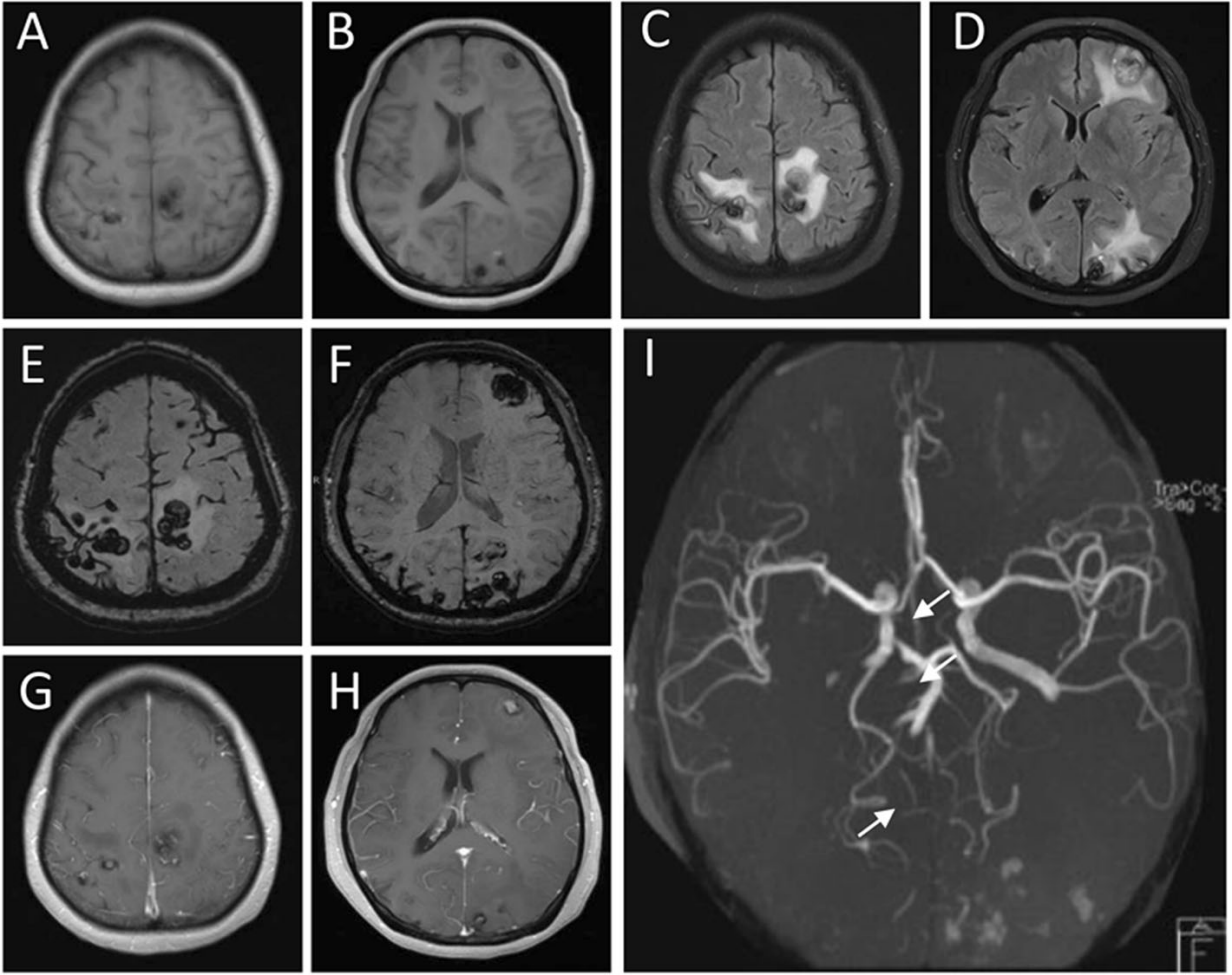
MRI brain images show multiple brain metastases of myxoma, hemorrhage and cerebral aneurysm formation from myxoma. a-b The axial T1-weighted images. c-d The axial T2-weighted flair images show multiple nodules or mass shadow. e-f The axial SWI images shows multiple cerebral hemorrhage. g-h Magnetic resonance enhanced scan showed heterogeneous reinforcement. i The MRA images for aneurysms
